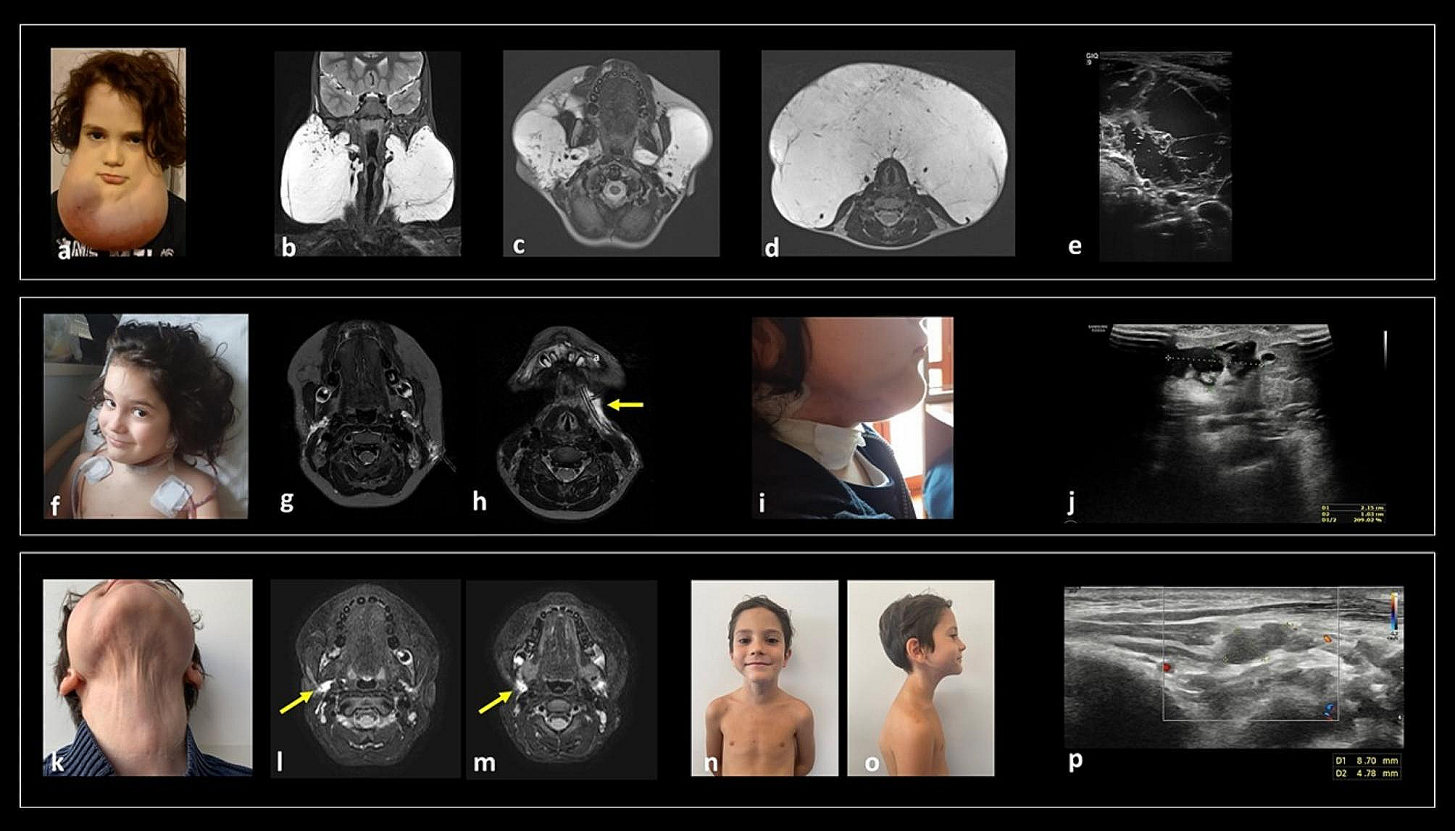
A giant swelling of a soft-elastic consistency originating from the subcutaneous tissues of the mandibular angles bilaterally with downward extension anteriorly to the neck was evident at 6 years and 4 months. The overlying skin showed clinical signs of infection (a). MRI (b-d) and US (e) revealed a large bulky macro-cystic mass in the suprahyoid and infrahyoid neck involving bilaterally the submandibular, parapharyngeal, parotid and vascular spaces, without mediastinal extension and determining minimal deformation of pharyngeal air column, which maintained regular patency. After surgical debulking, the clinical examination revealed an apparent complete response to treatment (f). MRI showed grossly total excision of the LM with minimal fluid collections in the submandibular spaces (g, h). The arrow indicates the left drainage tube in the submandibular space. Swelling in the retromandibular right angle and submental region was progressively documented after removal of drains (2 weeks post-surgery) (i, lateral view). The US performed at that time revealed slight enlargement of some cystic components in the submandibular and submental spaces (j). Clinical evaluation at last follow-up (during Alpelisib treatment at 125 mg/day) documented no evidence of swelling mass; the skin in the submental area and in the superior part of the neck appeared thin but not atrophic (k). Frontal (n) and lateral (o) view of the patient confirmed the absence of persistent swelling. The clinically detected facial asymmetry involved the subcutaneous tissues, probably because of the surgical procedure. MR (l, m) and US (p) showed small residual cystic component in the posterior portion of the right submandibular space (arrow in l, m)
